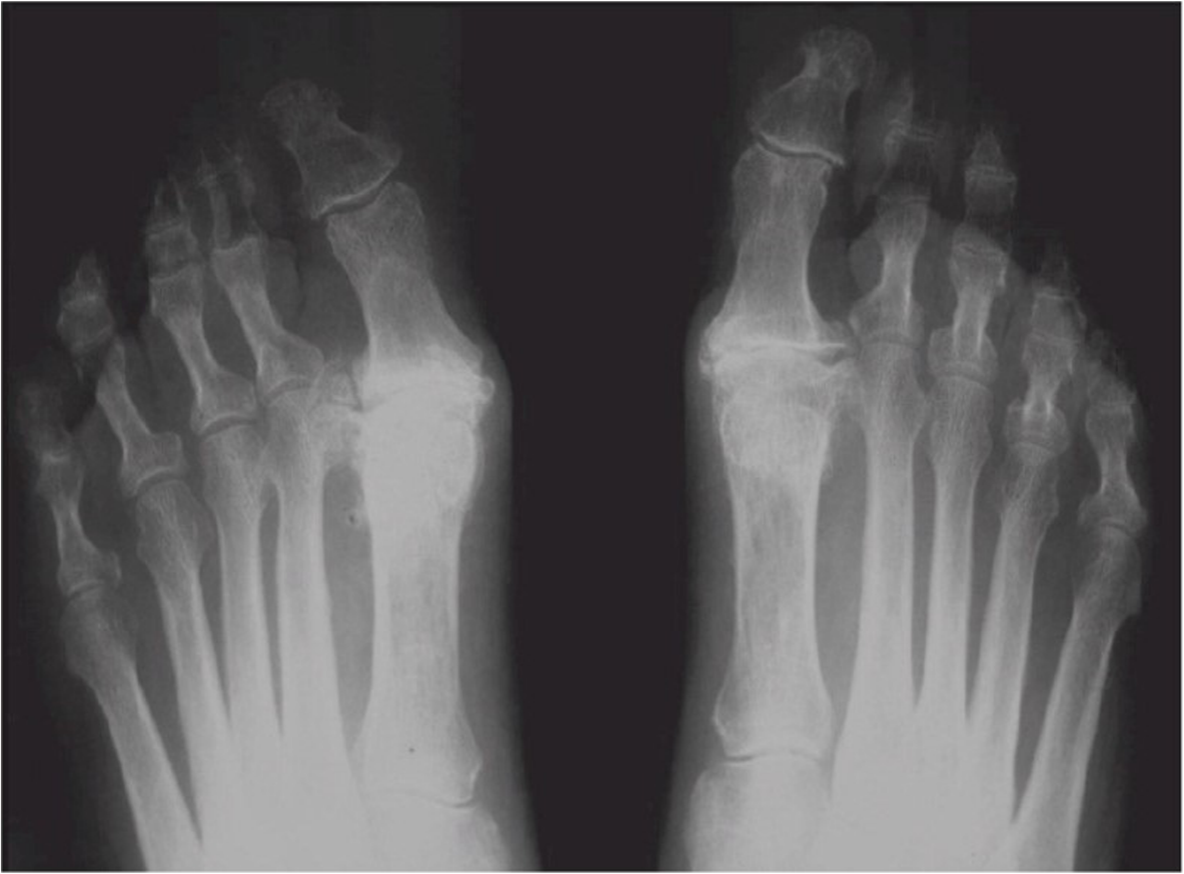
A standing X ray radiograph of a patient with bilateral hallux rigidus that was considered as severe
